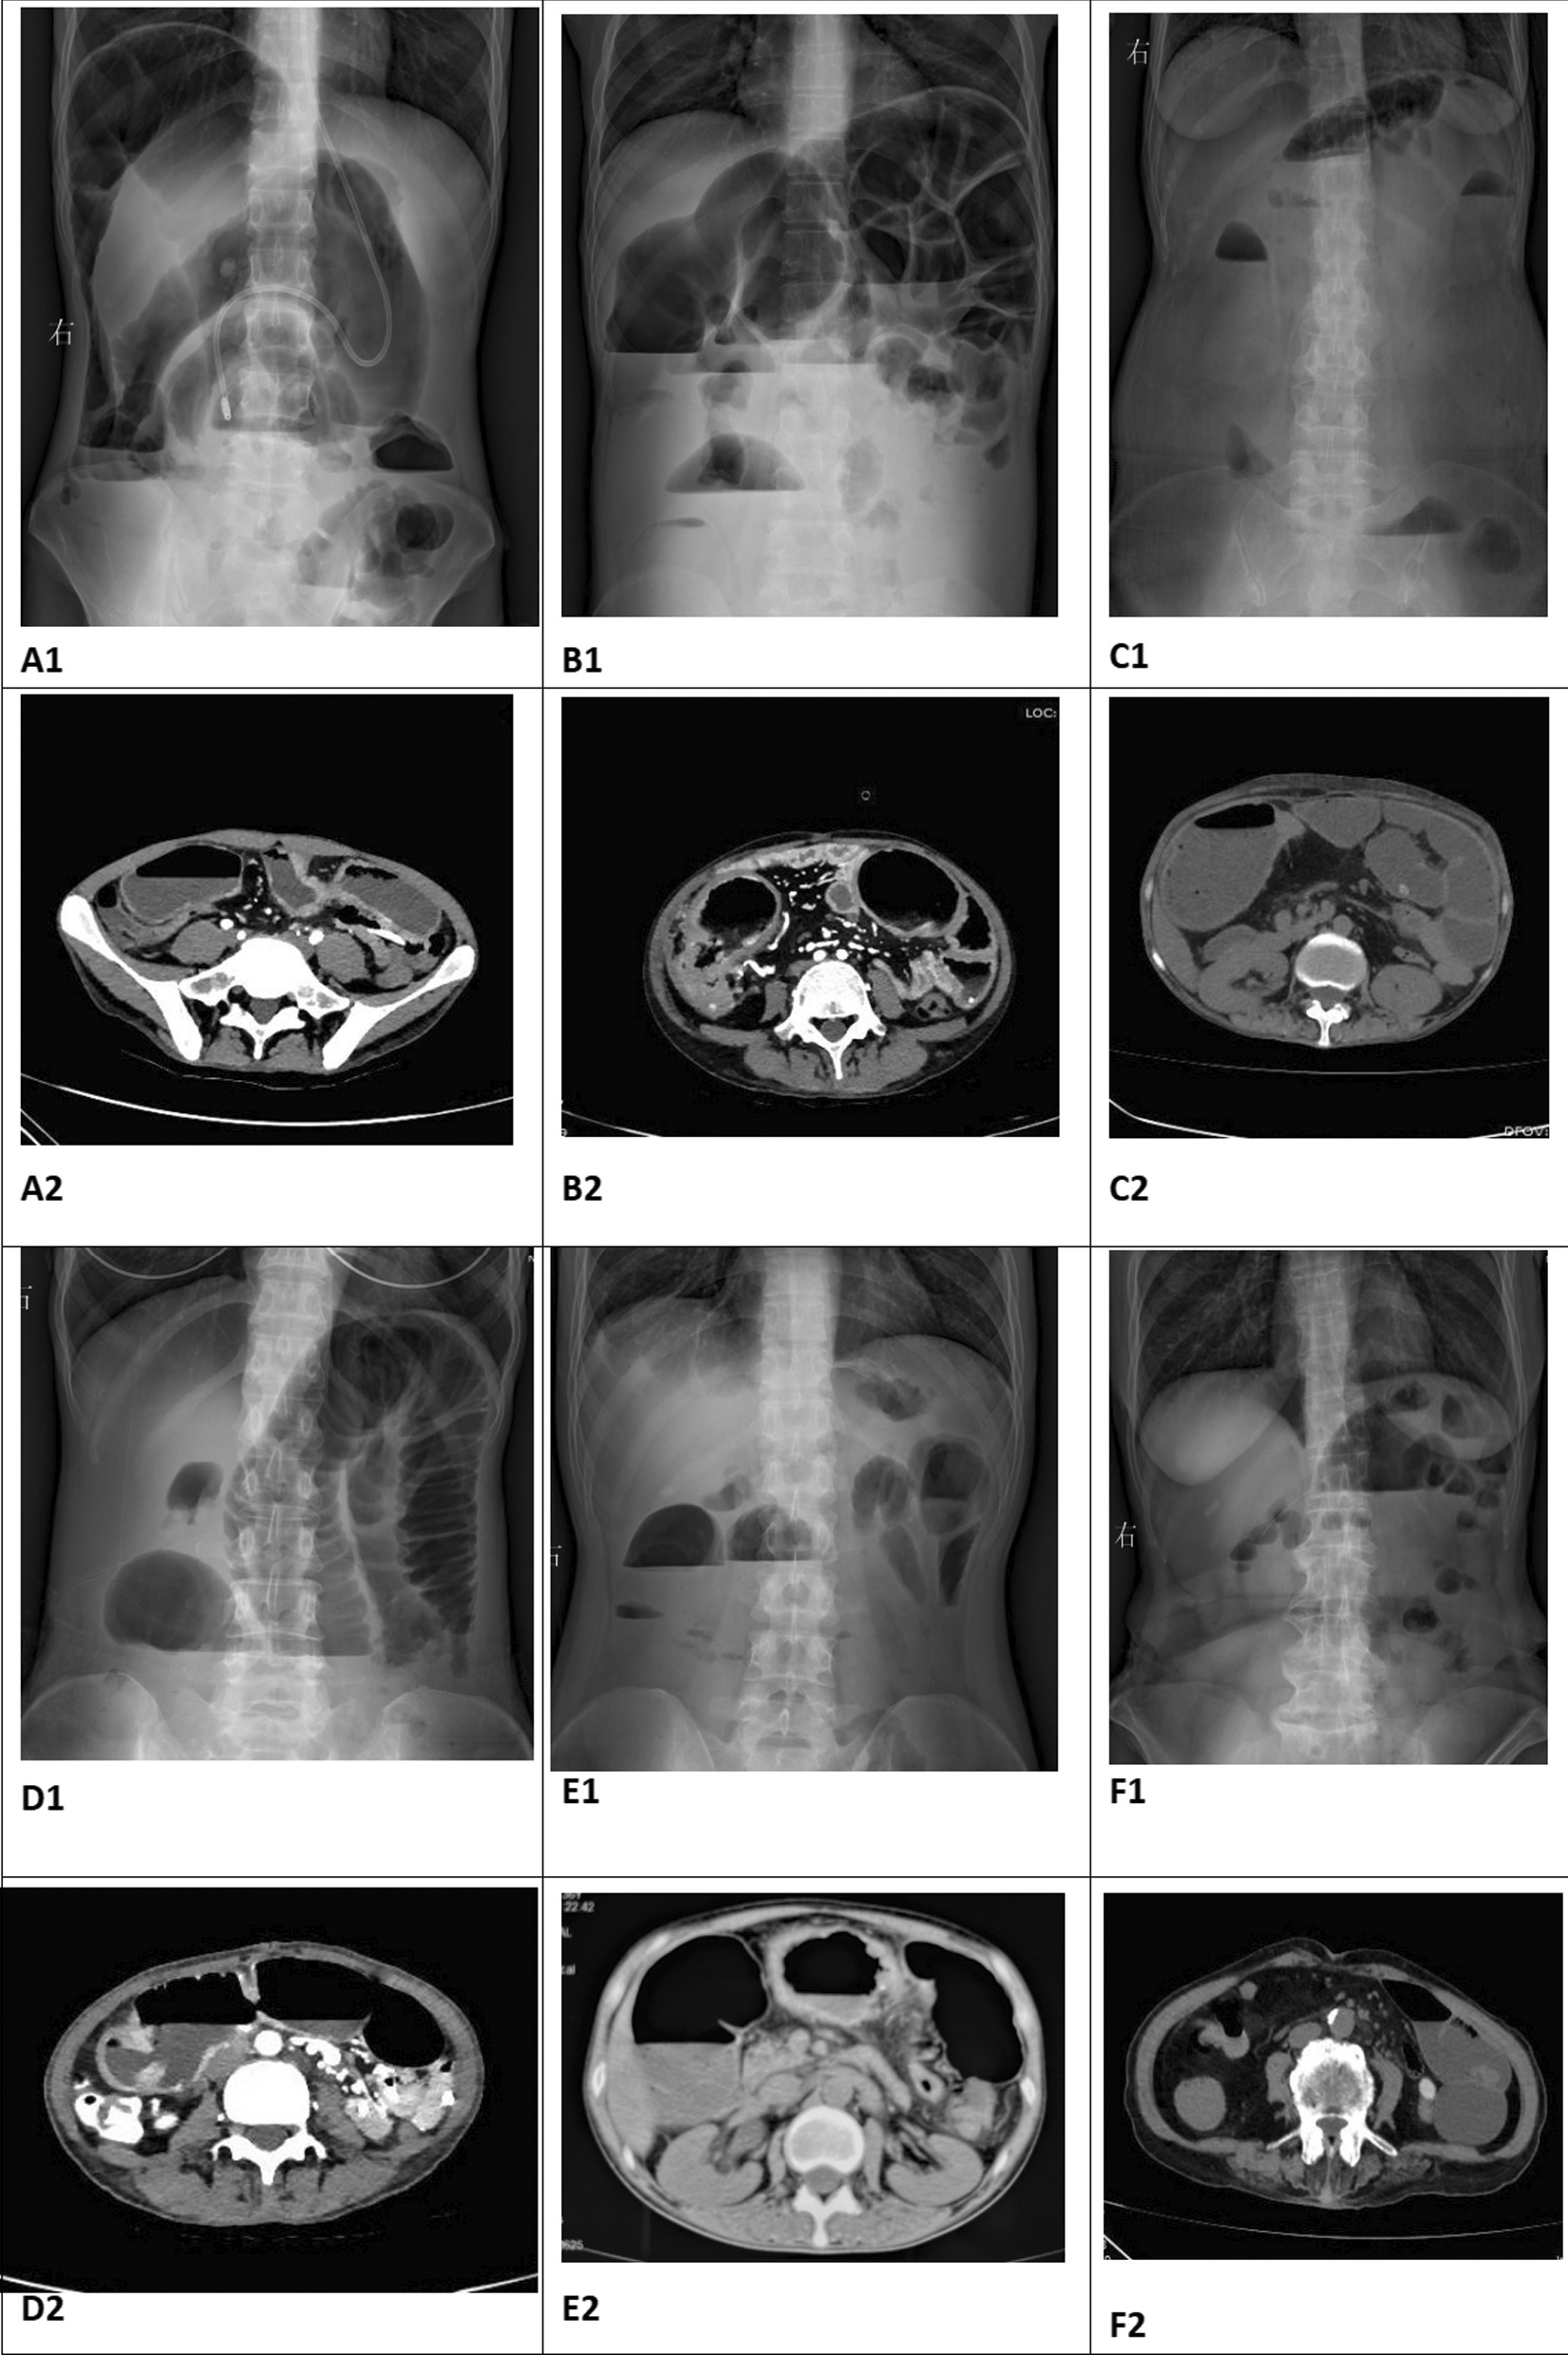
Abdominal X-Ray and CT at the beginning of obstruction confirmation. A1 and A2 showed the obstruction sign before tube insertion of patient 1; B1 and B2 showed the showed the obstruction sign before tube insertion of patient 2; C1 and C2 showed the obstruction sign before tube insertion of patient 3; D1 and D2 showed the obstruction sign before tube insertion of patient 4; E1 and E2 showed the obstruction sign before tube insertion of patient 5; F1 and F2 showed the obstruction sign before tube insertion of patient 6
